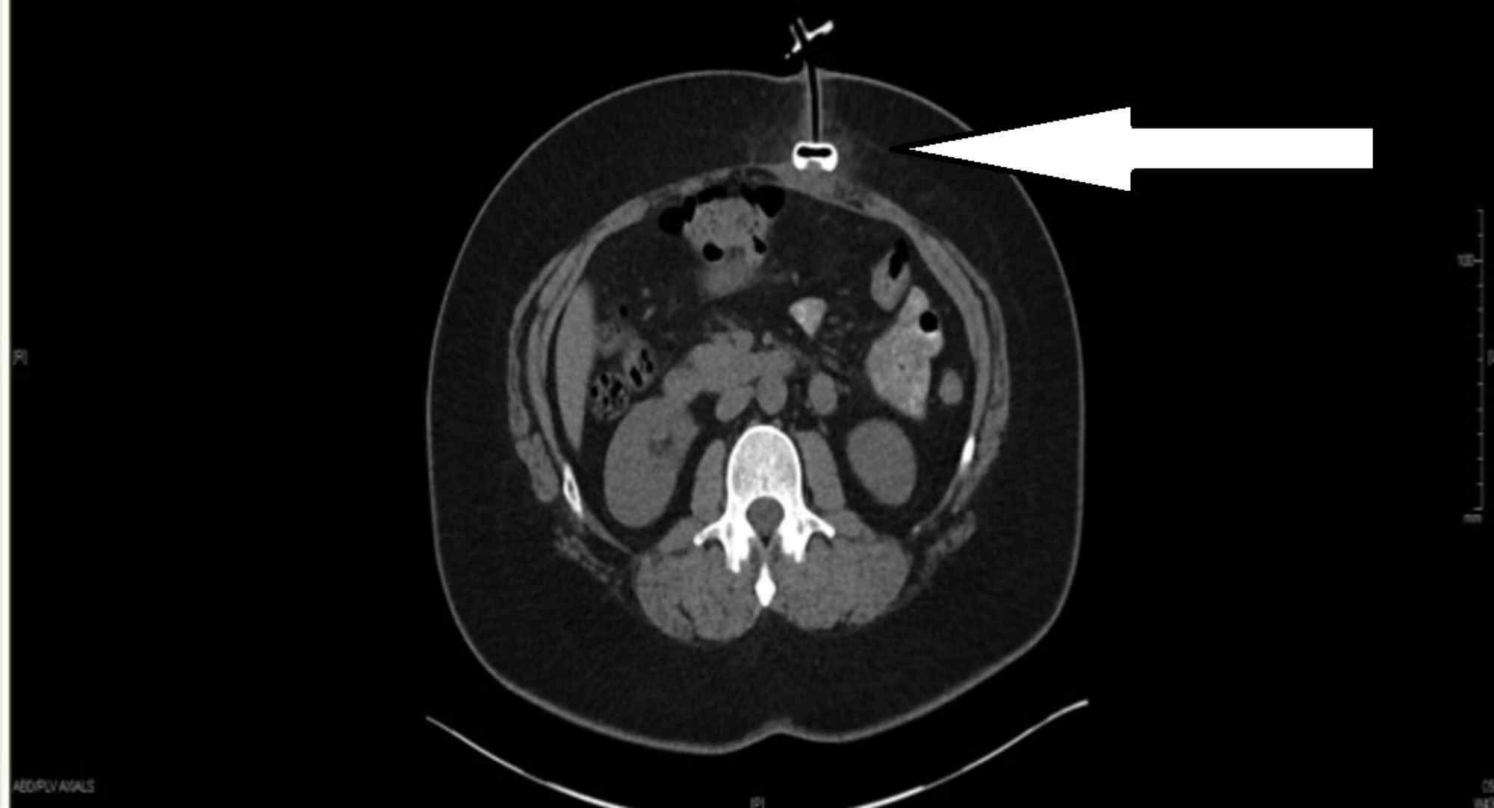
CT scan of the abdomen without contrast showing retraction of the internal bolster of the percutaneous endoscopic gastrostomy tube (solid white arrow) into the subcutaneous fat adjacent to the gastric wall.
